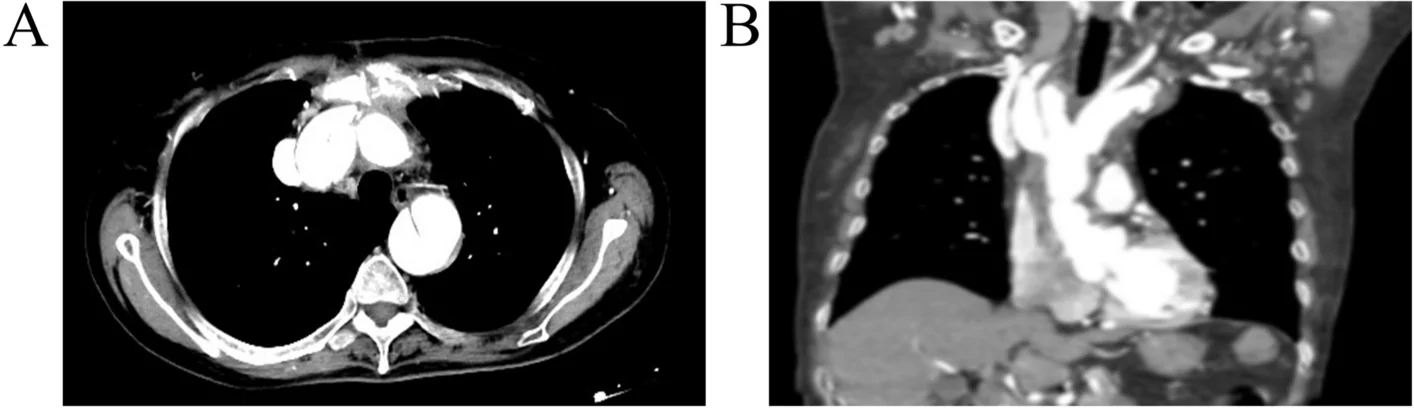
A, B CTA at 1 month postoperatively shows a newly developed dissection involving the proximal right aortic arch, without involvement of the anastomosis
